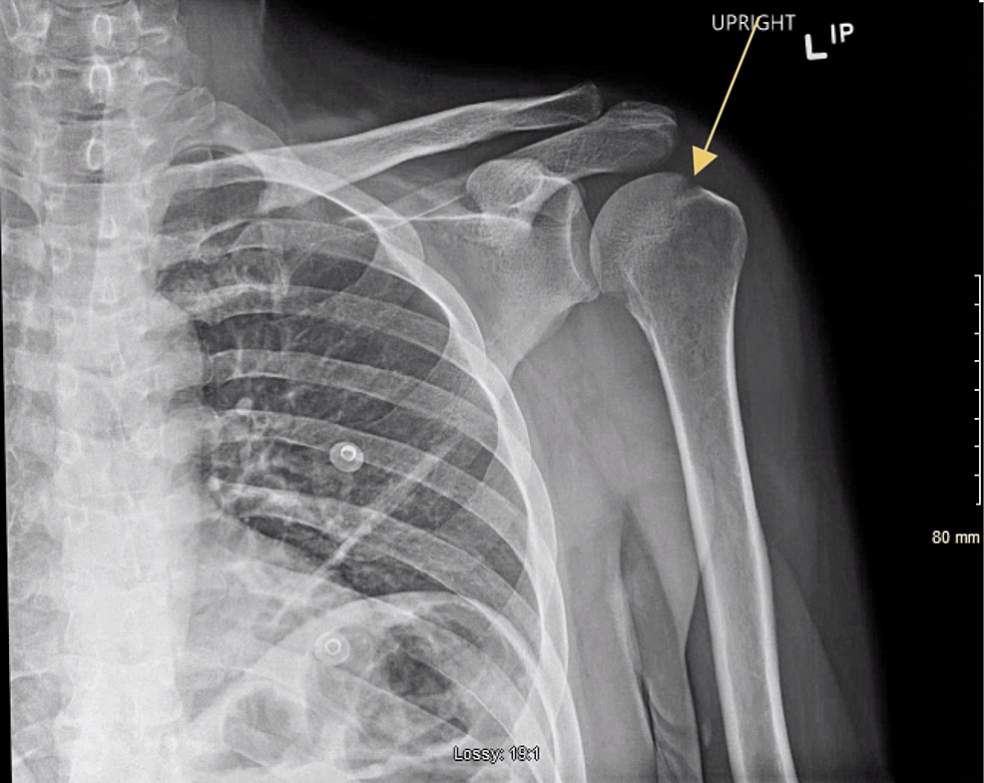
Radiographic findings of the left shoulder Description: Anterior-posterior shoulder radiograph demonstrating joint effusion with focal erosion of the left humeral head (yellow arrow)
